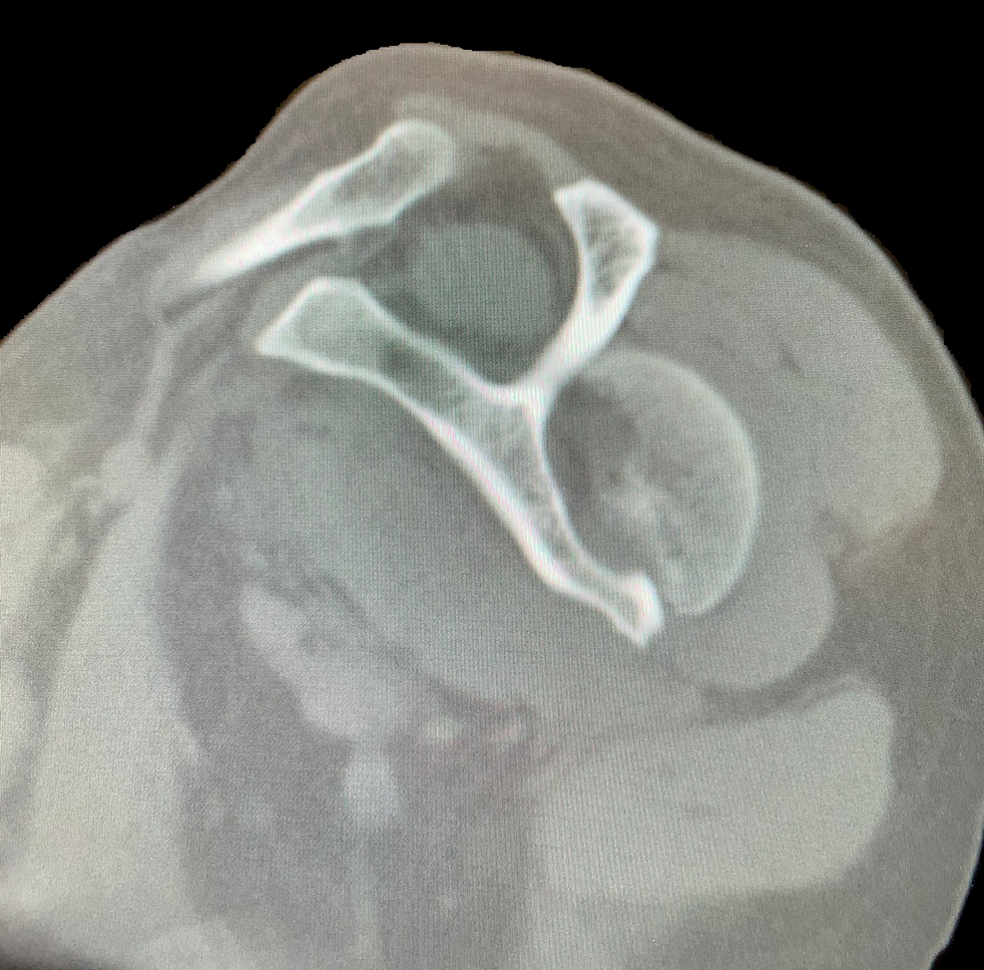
Sagittal view of preoperative CT scan of the right shoulder demonstrating a four-part proximal humerus (PH) posterior fracture-dislocation.
